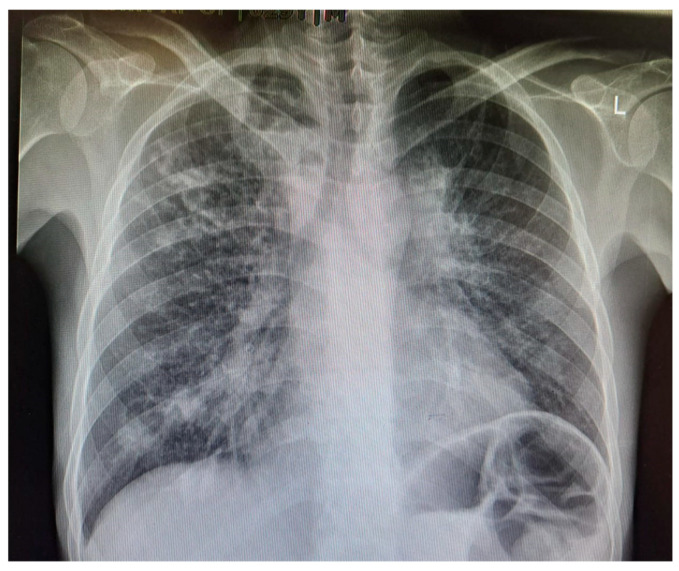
Chest X-ray of a 29-year-old male with active TB, showing bilateral upper lobe opacities and cavitation, consistent with advanced pulmonary TB. These findings reflect extensive lung parenchymal damage due to the infection.
